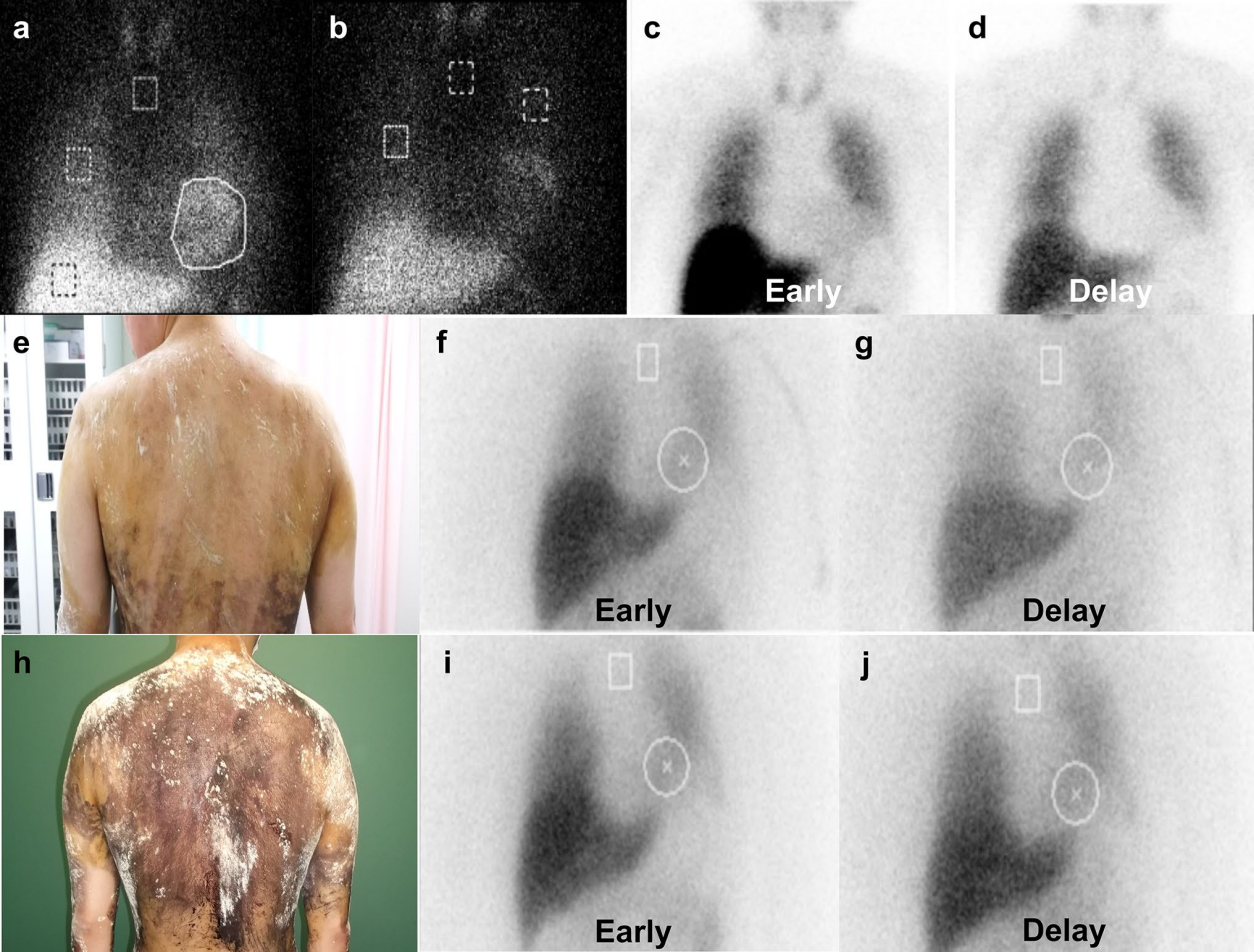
^123^I-MIBG myocardial scintigraphy and thermoregulatory sweating test. a–d, f, g, i, j Iodine-123 and meta-iodobenzylguanidine myocardial scintigraphy of Cases 1–3. Note the reduced heart-to-mediastinum (H/M) ratio: a early 1.94, b delayed 1.70 in Case 1; c early 1.53, d delayed 1.44 in Case 2; and f, g pre-immunotherapy: f early 1.49, g delayed 1.39 and increased washout rate (46.2%) in Case 3. i, j Post-immunotherapy. Note the slight improvements in the H/M ratio: i early 1.66, j delayed 1.63 and washout rate (28.5%). e, h Thermoregulatory sweating test in Case 3. e Pre-immunotherapy. Note the reduced sweating. h Post-immunotherapy. Note that generalized sweating was apparent
